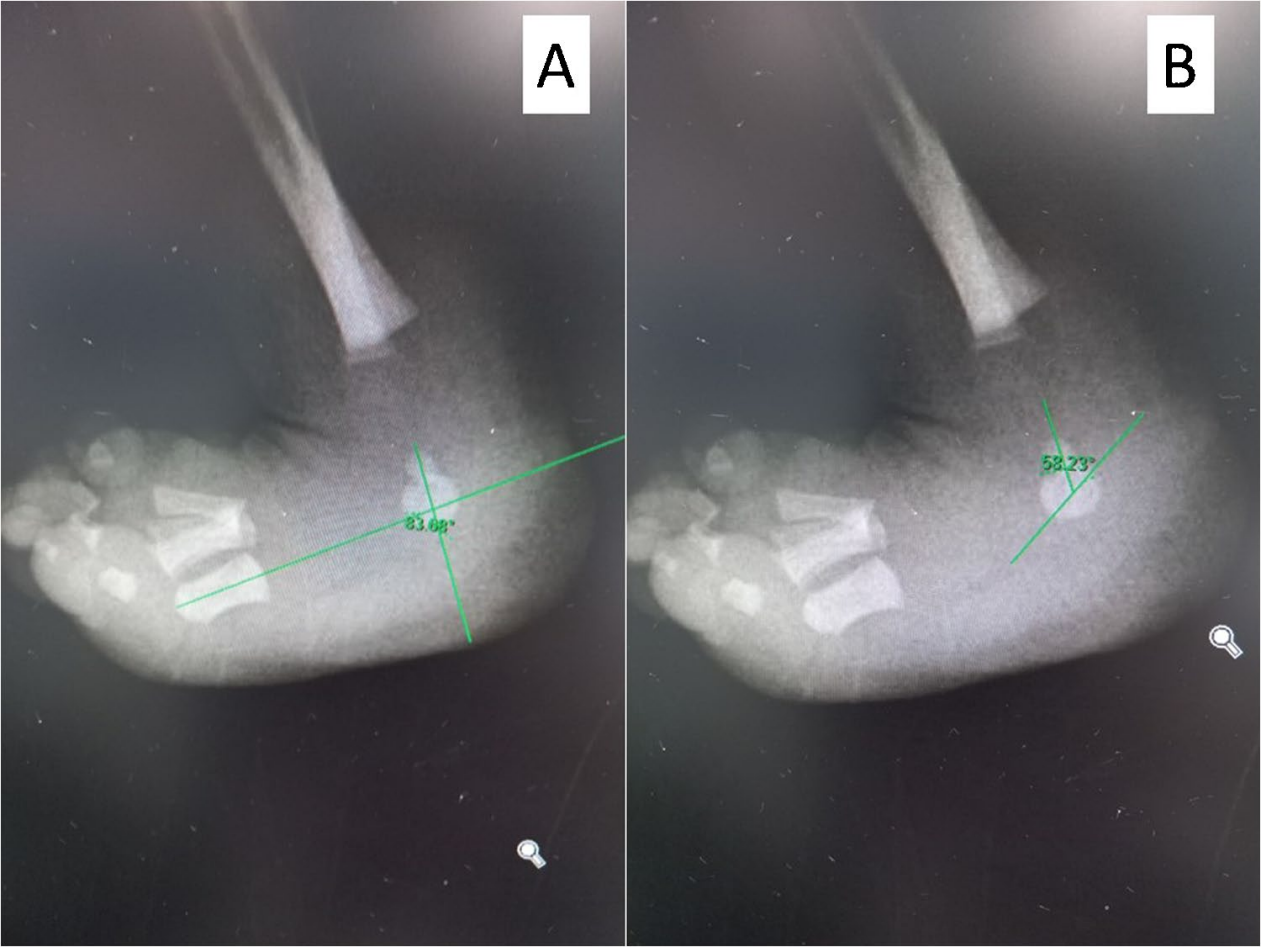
Xray outlining the angles measured, TAMBA (A) and the talocalcaneal angle (B)
